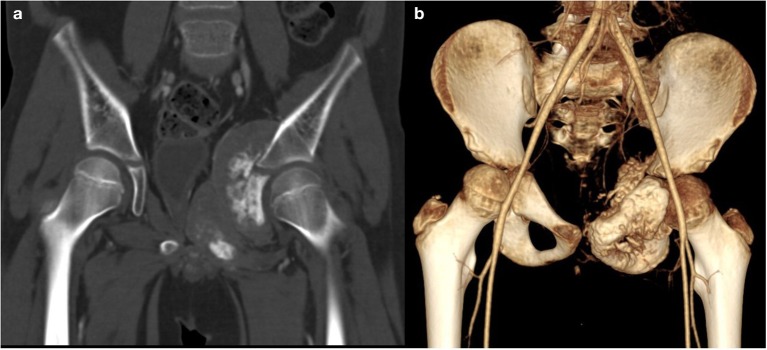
CT. Coronal (a, bone window) and 3D (b) reconstruction show a voluminous tumor involving the left ischio-and ileo-public branches with extensive bone destruction, speculated periosteal reaction, and partially mineralized soft tissue mass.
